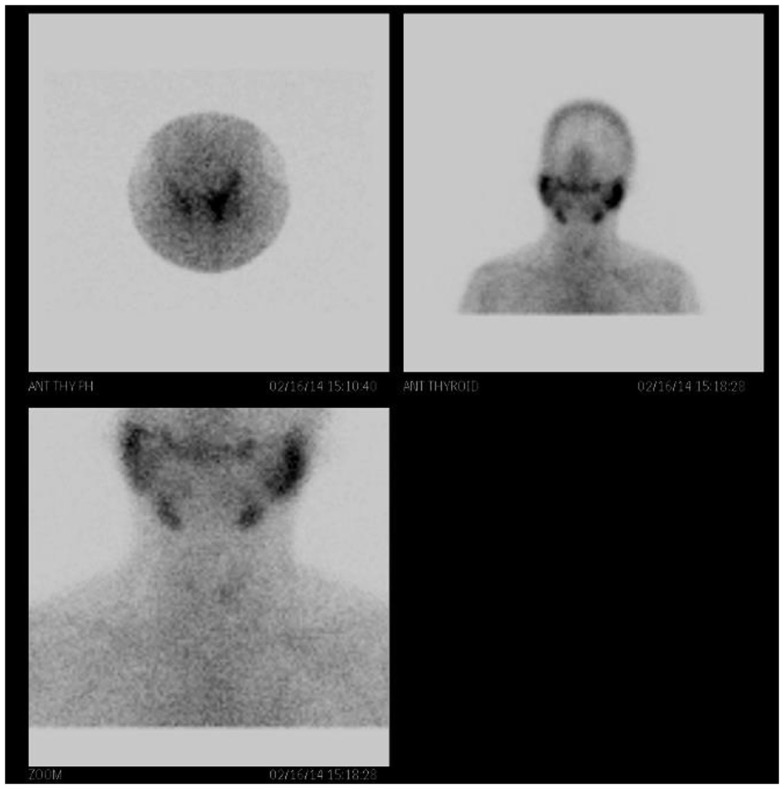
Technetium-99m (Tc-99m) pertechnetate scintigraphy of the patient’s thyroid gland is faintly observed with generalized reduced tracer uptake in the thyroid gland. There is increased background tracer activity in the salivary glands. These findings are compatible with thyroiditis.
